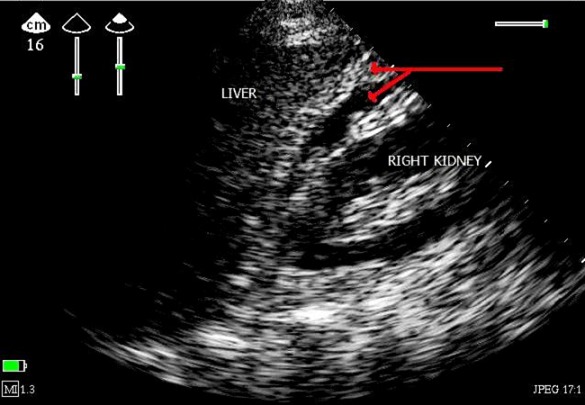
Abdominal ultrasonography mode 2 D showed a effusion at the Morrison's pouch with low abundance and contusion of the lower edge of the liver (red arrow)V
